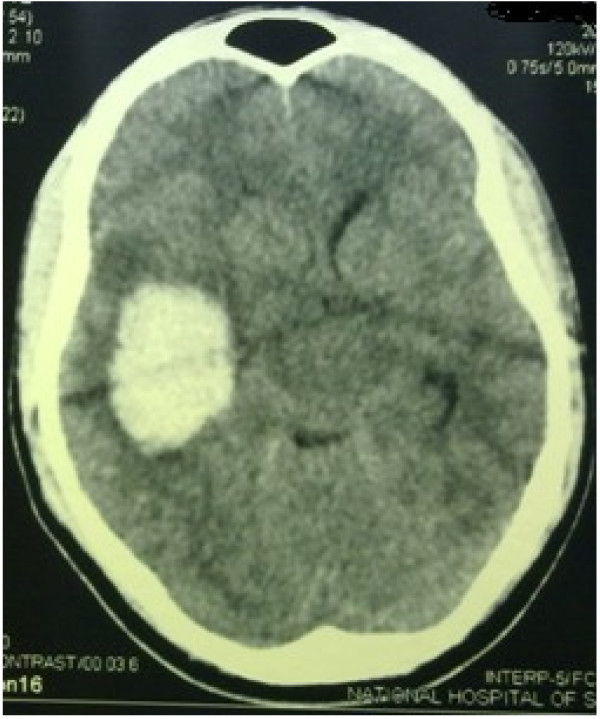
Non-contrast CT brain showing a massive left temporo-parietal region intra-cerebral haemorrhage.
